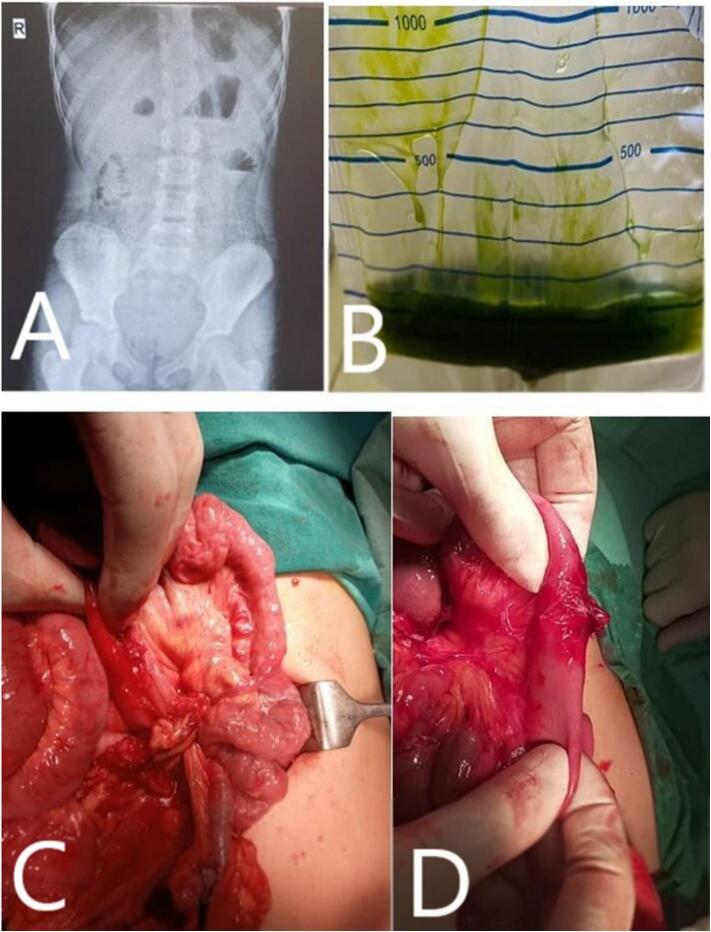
Radiological, clinical, and intraoperative findings. (A) Plain abdominal X-ray showing multiple air-fluid levels suggestive of small bowel obstruction. (B) Greenish bilious aspirate obtained via nasogastric decompression. (C and D) Intraoperative images showing dense inflammatory adhesions between small bowel loops without prior surgical history, consistent with a primary adhesive process.
